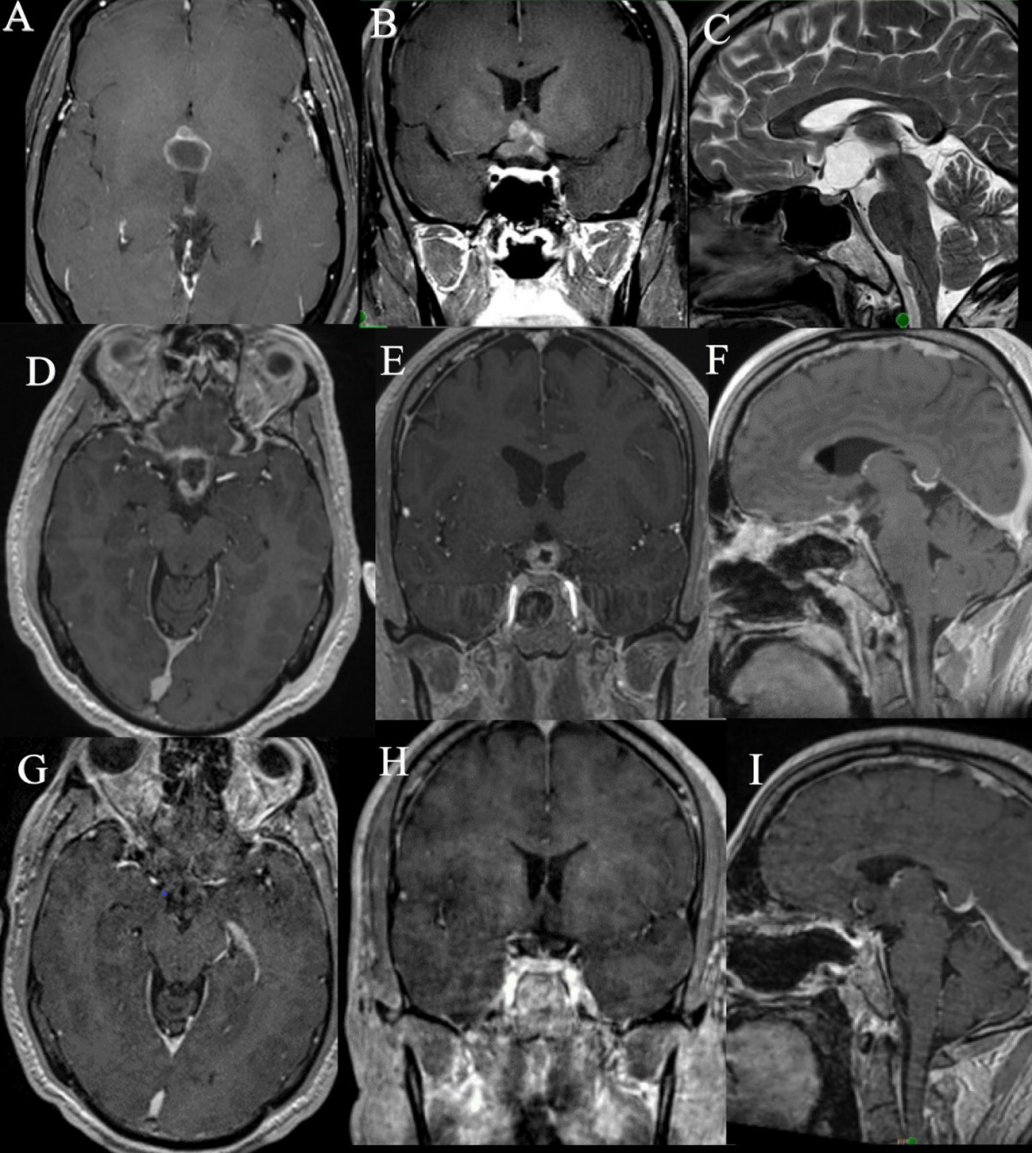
MRI images of Case 2: (A–C). Preoperative MRI images, (D–F). Immediate postoperative MRI images showing the residual tumor, (G–I). MRI images obtained after 19 months of dabrafenib, trametinib therapy showing significant reduction in size of tumor.
